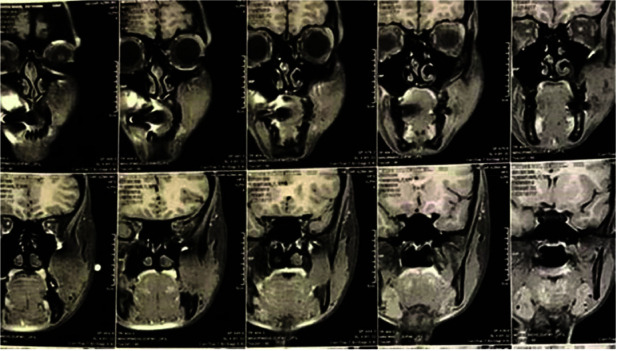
Post-operative MRI scans
